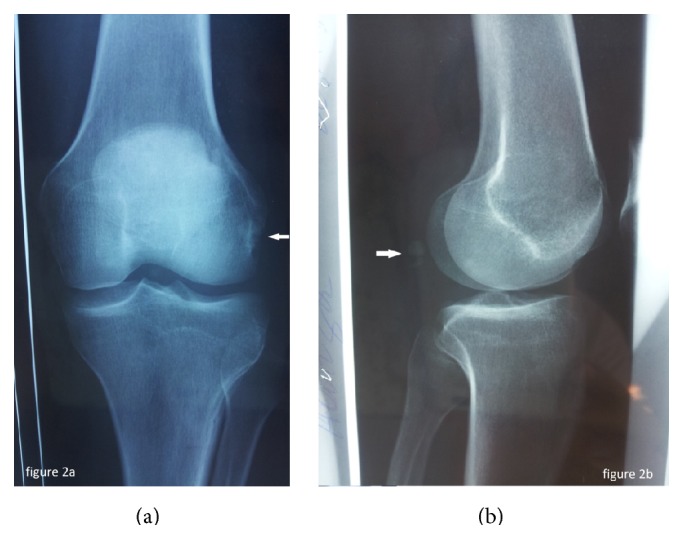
Anteroposterior (a) and lateral (b) plain films of the left knee showing a fabellar fracture.
